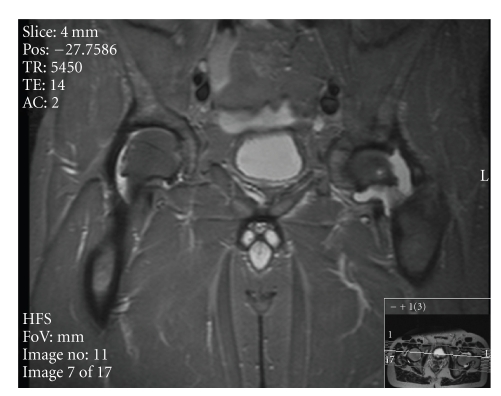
T2 weighted MRI scan of the pelvis.
